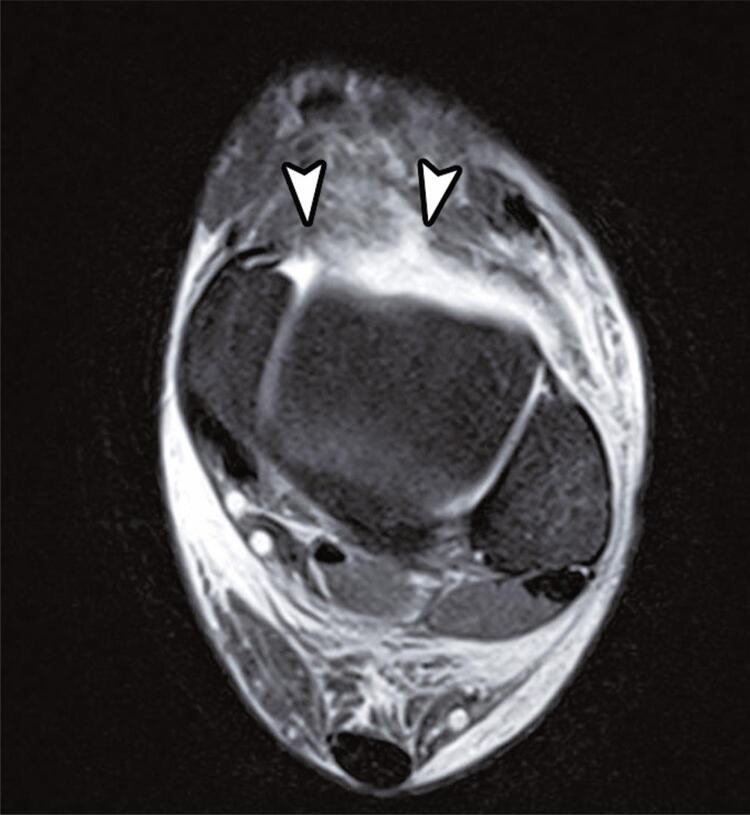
Axial T2-weighted fat-suppressed magnetic resonance image shows extensive edema involving the extensor retinaculum, along with areas of discontinuity of the fibers (arrowheads)
